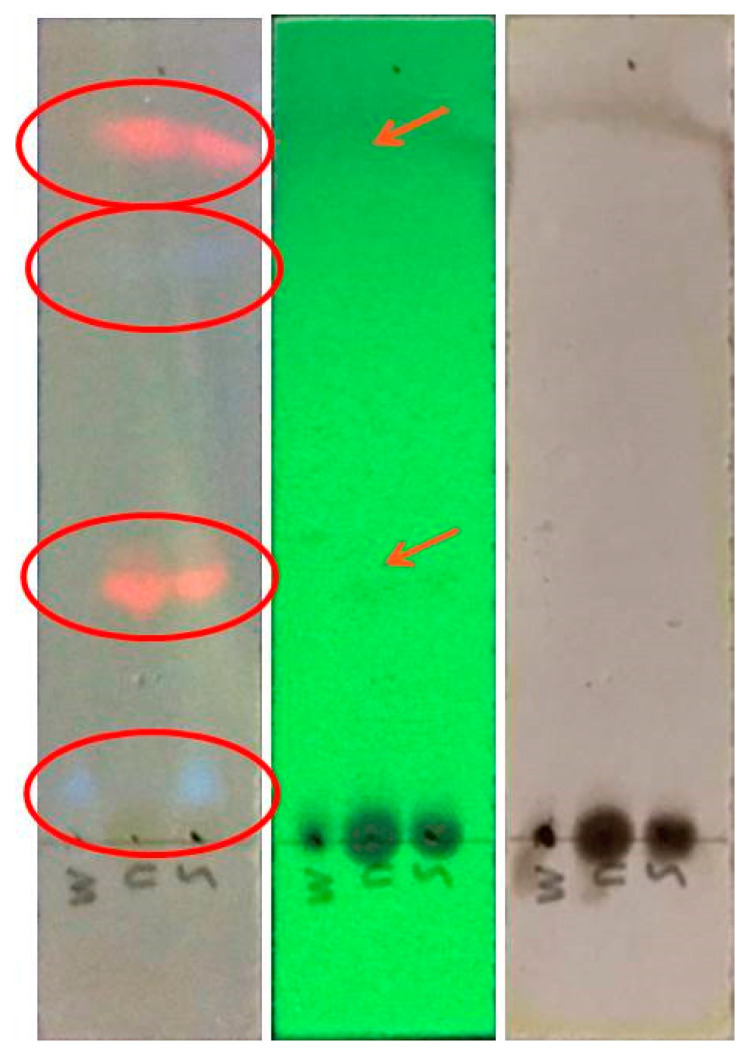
TLC chromatogram under Visible light (370 nm), UV light (254 nm), and dyed with Para-anisaldehyde, from left to right. M = Microwave-assisted extraction; U = Ultrasound-assisted extraction; S = Solvent extraction. Red circle and arrow indicates the presence or absence of peaks.
